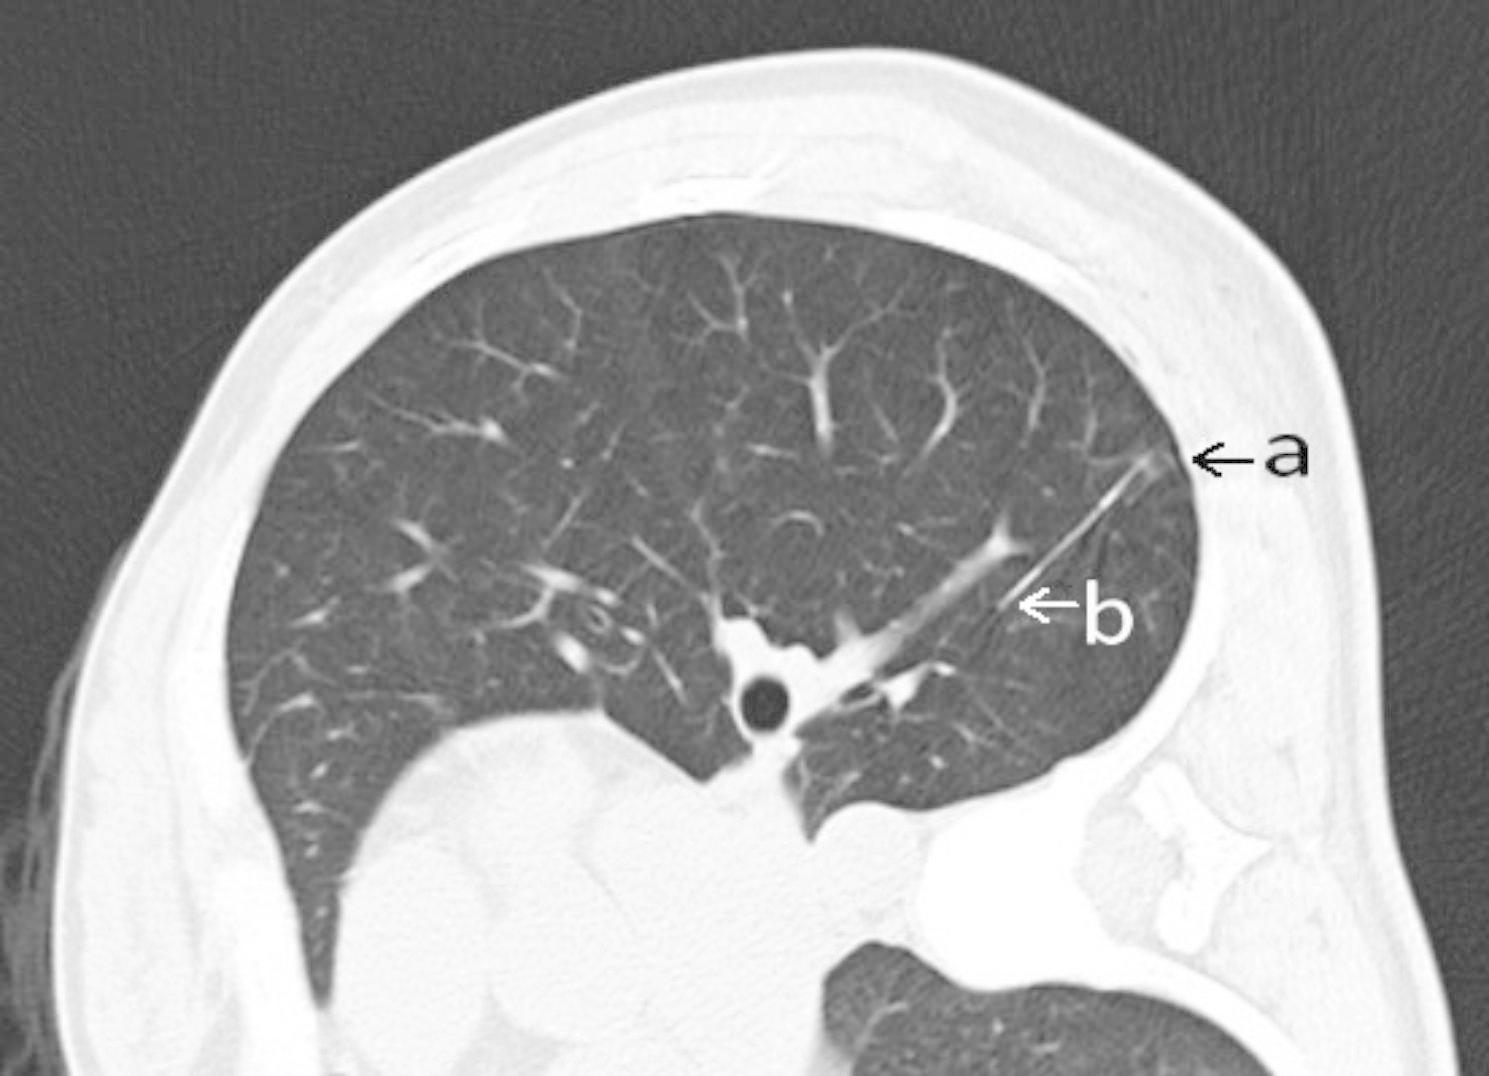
Images of lung CT scan for the distance between the lesion and the wire tip. As shown in the image, there was a distance of more than 2 cm between the lesion and the tip of the wire. (a: lesion, b: tip of the wire)
